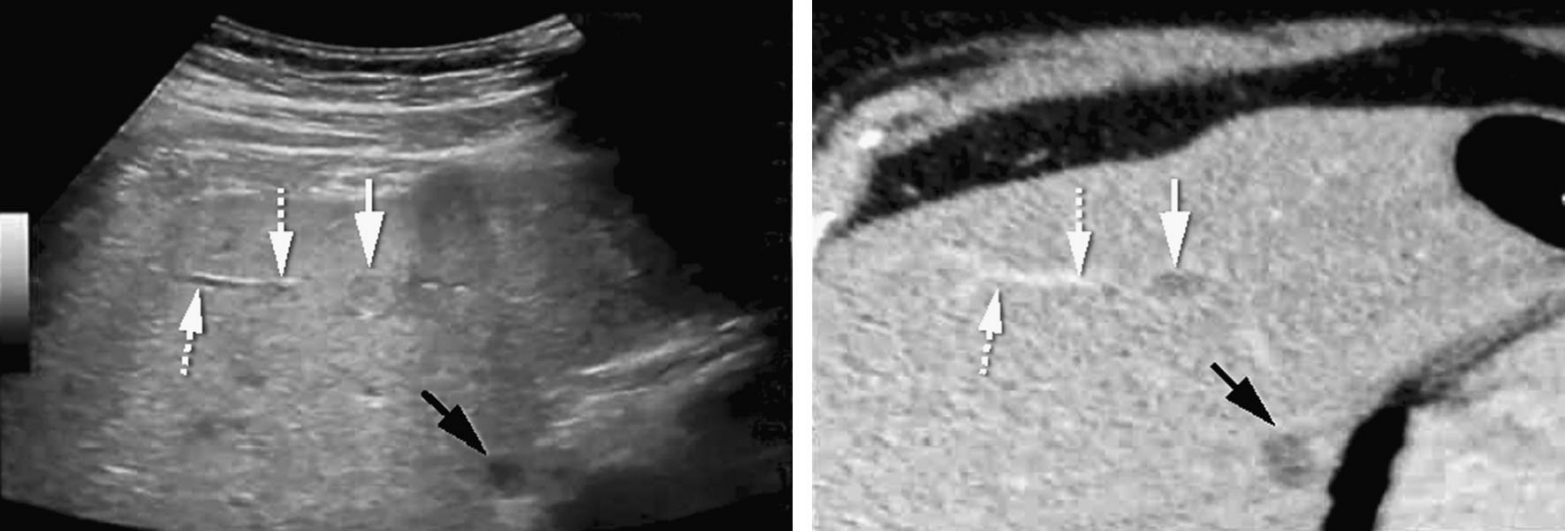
Example of automatic co-registration of US and CT images in a 65-year-old male with colorectal liver metastases. Two sub-centimeter lesions were characterized as metastases with the use of MRI (not shown), but were not found on pre-procedural ultrasonographic examination. The patient was scheduled to undergo ablation using the GE Logiq E9 navigation system. CT with intravenous contrast was obtained with the OmniTRAX™ Active Patient Tracker attached to the patient. The images show adequate co-registration of US (*left*) and CT (*right*) with matching position of a portal vein branch (*dotted arrows*) and liver cyst (*black arrows*). After image fusion, the liver metastasis were vaguely seen (*white arrow*; second lesion not shown) and could be targeted with a radiofrequency probe. Post-ablation CT showed a good location of the ablation zone, and no recurrence has occurred during follow-up
